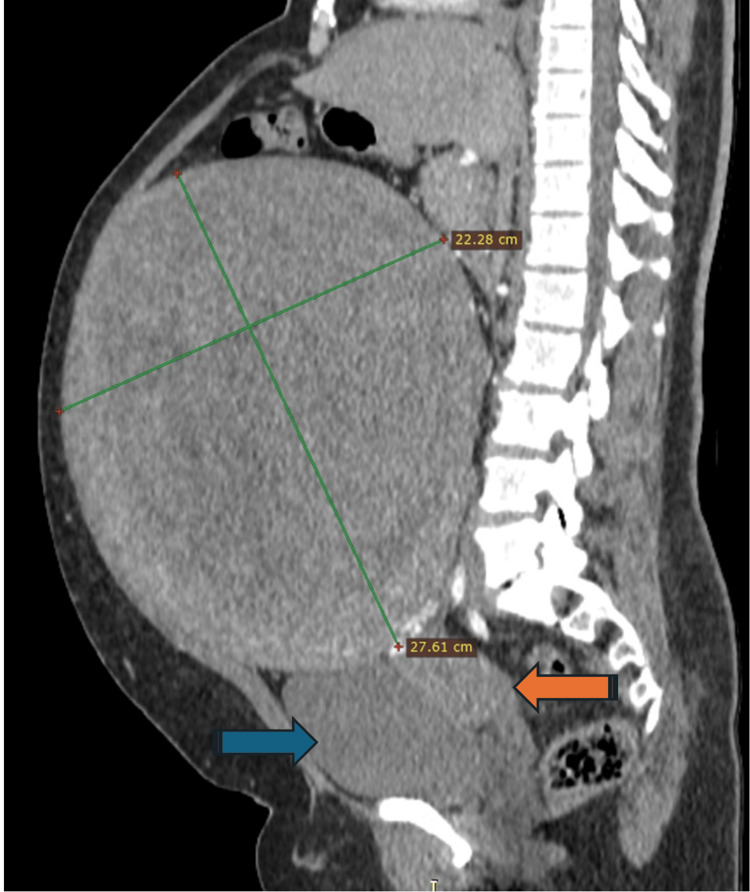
Contrast-enhanced sagittal CT demonstrates a giant uterine subserosal leiomyoma arising from the uterine fundus, measuring 27.61 × 22.28 cm. Blue arrow: urinary bladder and orange arrow: uterus.
